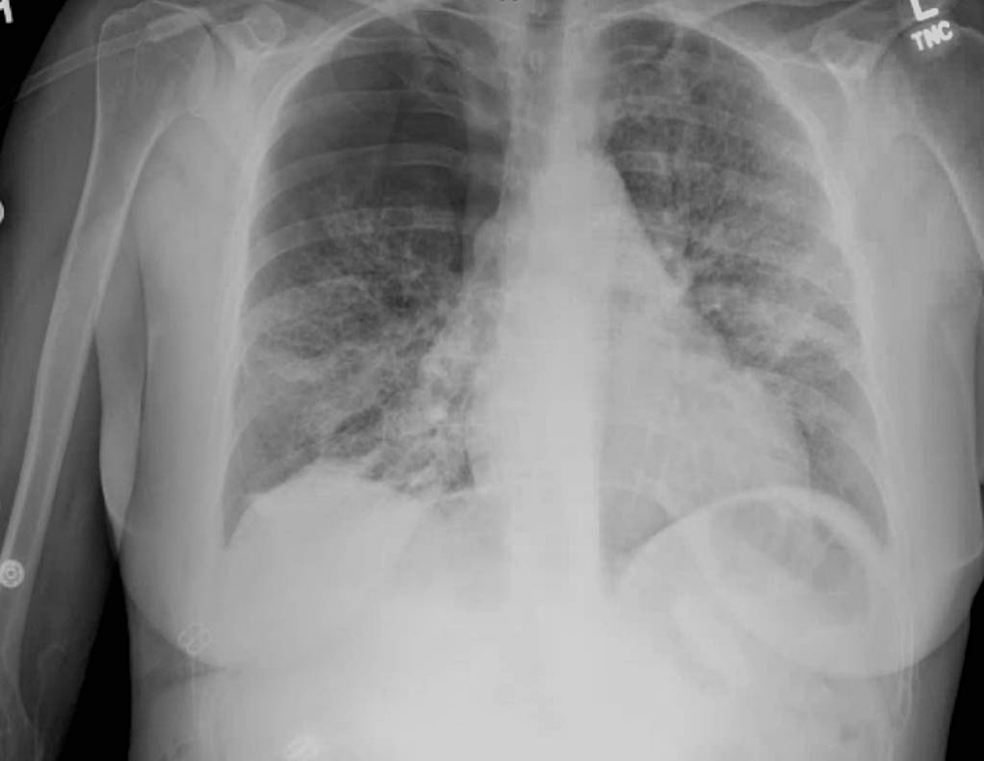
Chest x-ray showing right-sided pneumothorax and diffuse infiltrates.
